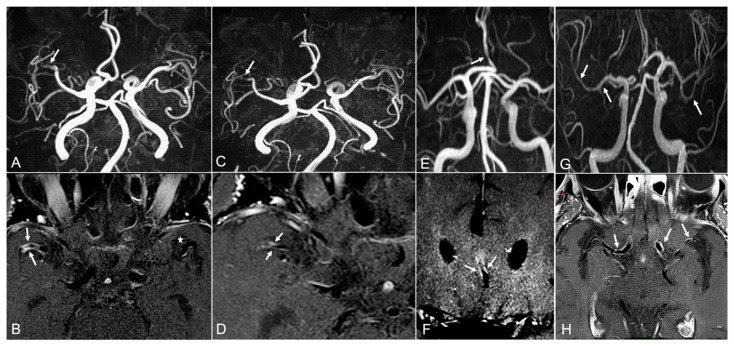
VWMRI findings in CNS vasculopathies. TOF-MRA of a patient with PACNS and recurrent ischemic strokes shows stenosis of M1 segment of MCA (panel (A), white arrow) with corresponding vivid concentric enhancement of arterial walls in post contrast in T1W black blood sequence (panel (B), white arrows) (* contralateral normal M1 segment). After treatment with intravenous high dose methylprednisolone and cyclophosphamide bolus, contrast enhancement was markedly reduced (panel (D), white arrows) with stable MRA findings (panel (C), white arrow). The patients had no recurrence of stroke during follow-up. MRA and VW-MRI findings of a patient with COVID19-associated cryptogenic ischemic stroke demonstrated stenosis of ACA (panel (E), white arrow) with corresponding contrast enhancement on VW-MRI (panel (F), white arrow). MRA of a patient with syphilitic CNS vasculitis revealed multifocal arterial lumen irregularities (panel (G), white arrows) and VW-MRI demonstrated multifocal enhancement of correspondent MCA segments (panel (H), white arrows). CNS: central nervous system; TOF: time of flight; MRA: magnetic resonance angiography; PACNS: primary angiitis of the CNS; MCA: middle cerebral artery; T1W: T1 weighted; VWMRI: vessel wall MRI; COVID19: Coronavirus disease 2019.
